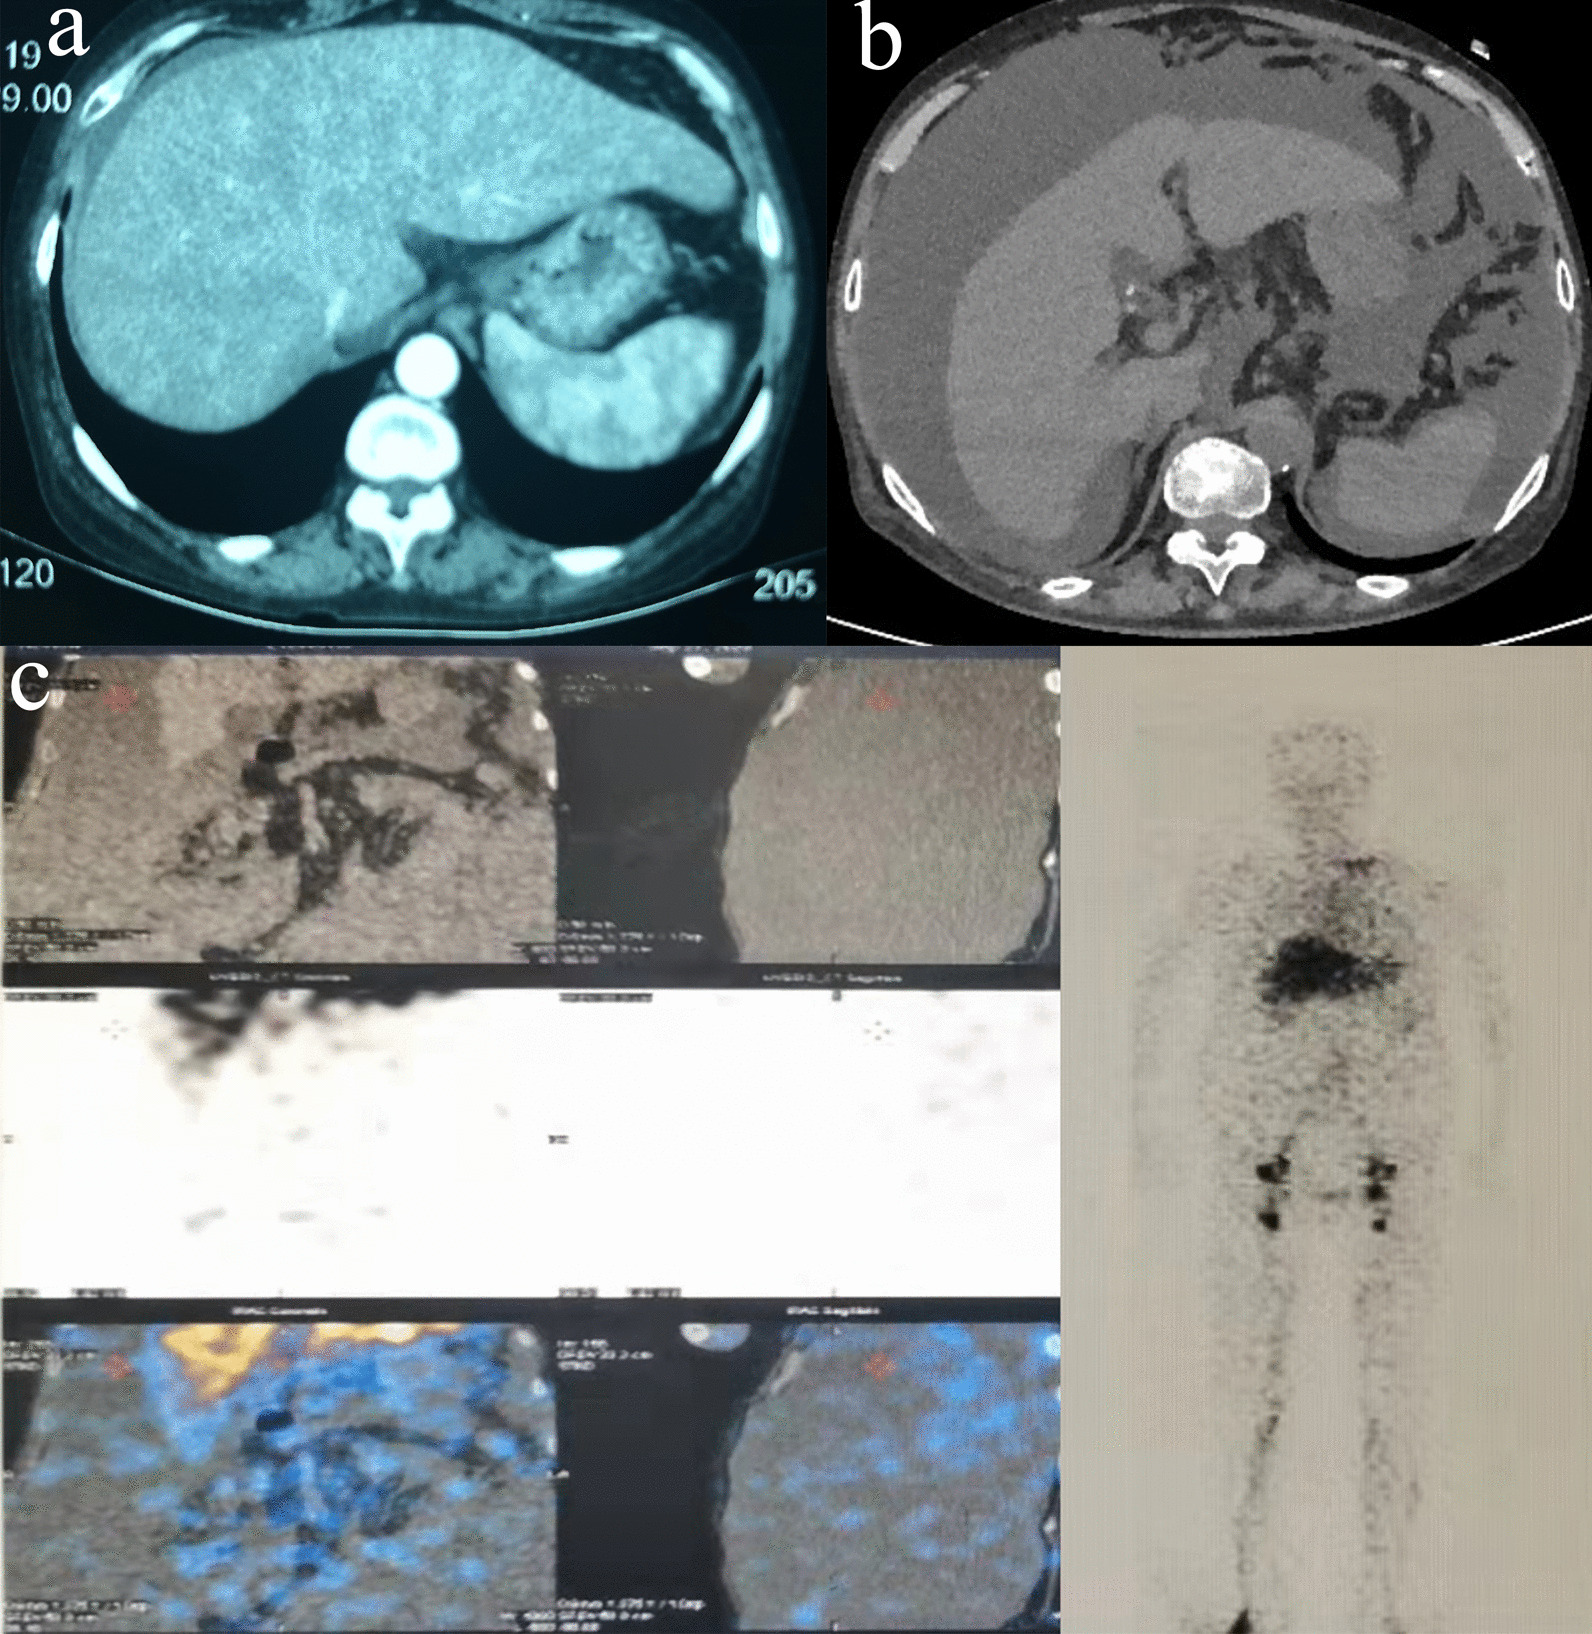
Imaging examinations. a Abdominal CT indicated a small amount of effusion around the liver before laparoscopic cholecystectomy; b Abdominal CT showed thickening of the peritoneum, a large amount of ascites, a decreased liver volume compared to pre-operation; c Lymphoscintigraphy revealed that a small amount of radioactive filling was found in the abdominal cavity
